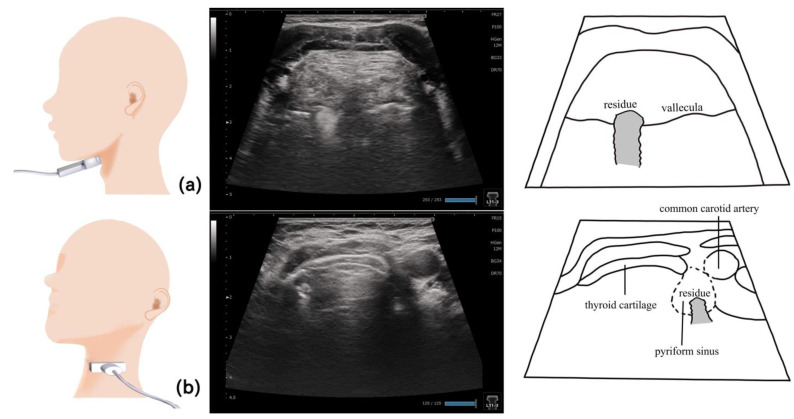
Pharynx in a dysphagic patient. A dysphagic patient showing residues in the vallecula (a) and pyriform sinus (b) on the ultrasound image.
